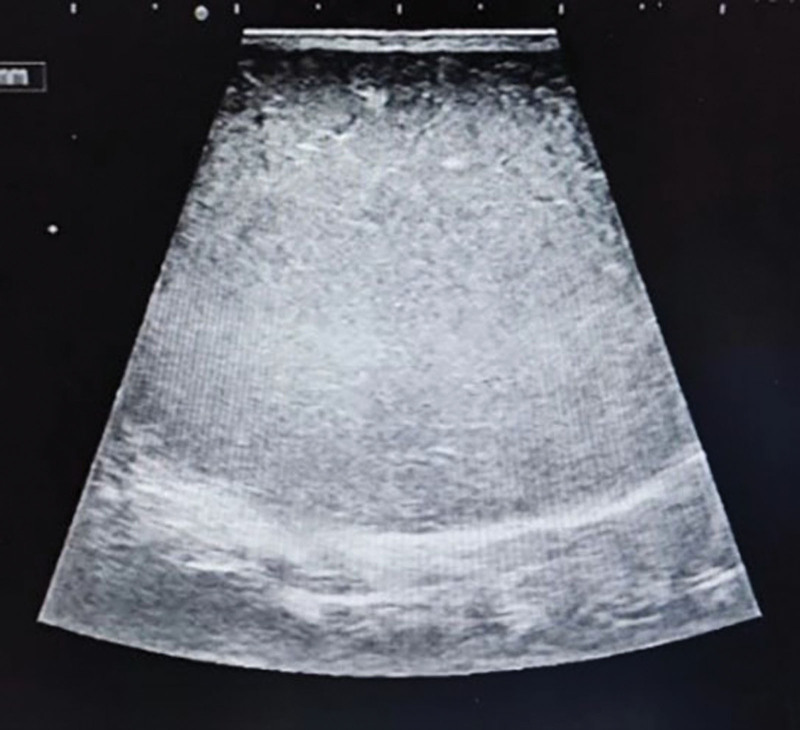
Ultrasound showed a cystic appearance, filled with punctate and flocculen.
